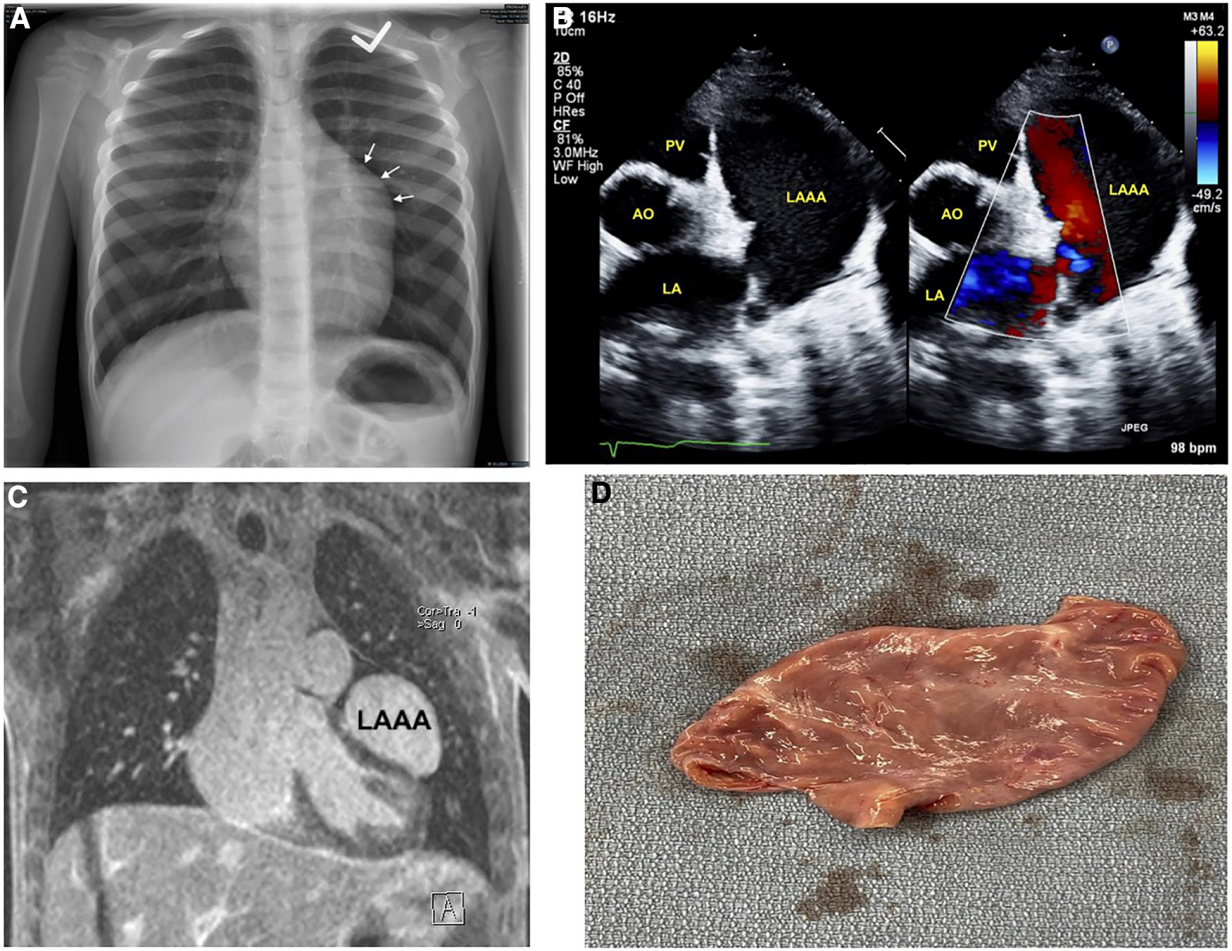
Imaging of left atrial appendage aneurysm. (A) Initial chest x-ray showing an unusual contour along the left heart border. (B) Transthoracic echocardiography short-axis sweep with colour doppler demonstrating a cavity adjacent to and potentially communicating with the left atrium. (C) Magnetic resonance imaging revealing the LAAA, and compressing the left ventricular anterior and anteroseptal walls. (D) Post-operative image of the resected LAAA measuring 5.9 × 3.1 × 1.9 cm. AO, aorta; LA, left atrium; LAAA, left atrial appendage aneurysm; PV, pulmonary valve.
